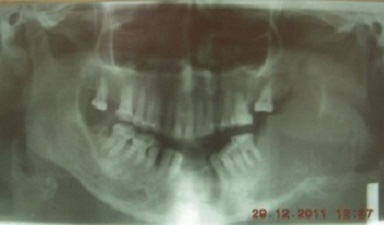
Orthopantomogram showing ill defined radiolucency associated with pathological fracture
